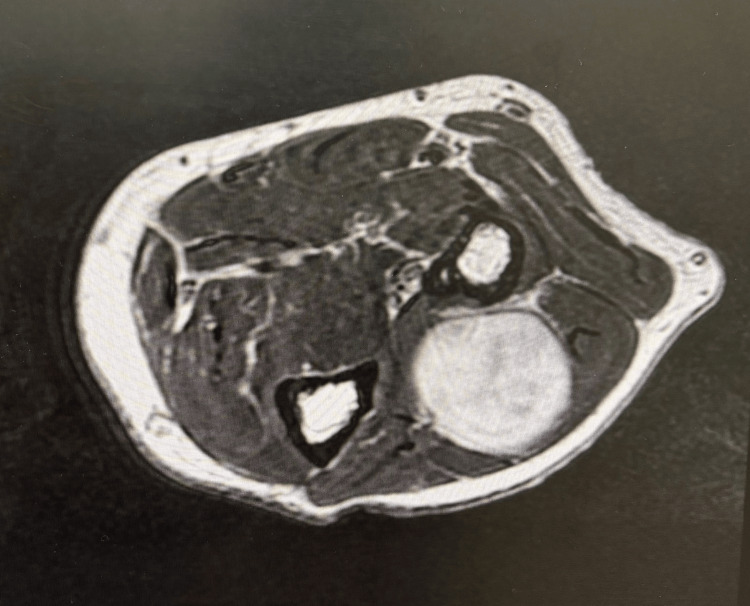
MRI axial section showing well-defined PIN schwannoma MRI: magnetic resonance imaging; PIN: posterior interosseous nerve
